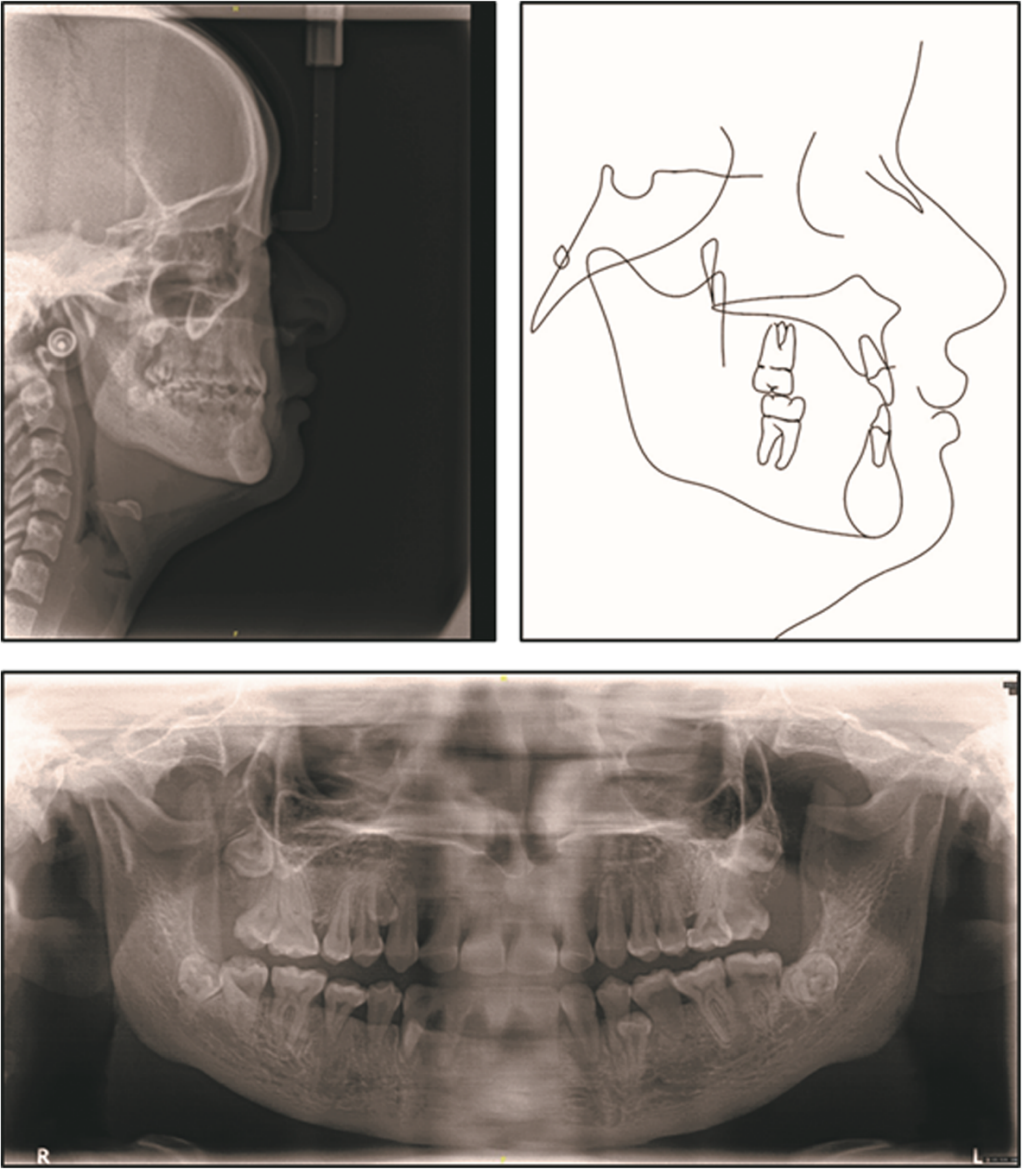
Post-treatment panoramic radiograph, lateral radiograph, and cephalometric tracing
